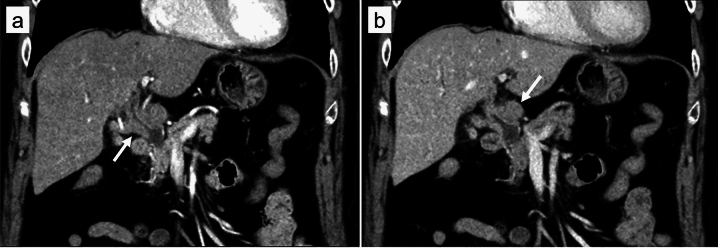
Contrast-enhanced computed tomography. a Image depicting thickening of the perihilar bile duct wall with contrast enhancement (arrow). b Image of an enlarged lymph node next to the perihilar bile duct (arrow)
